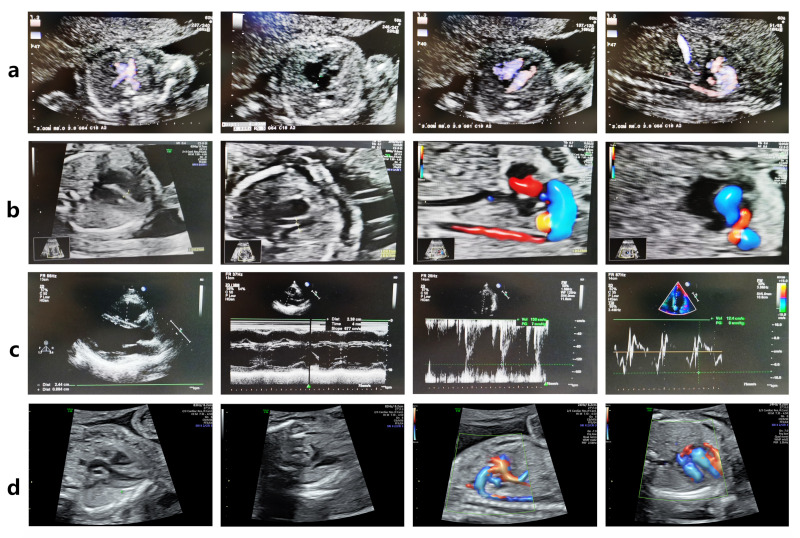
The *FLNC* variant leads to fetal Tetralogy of Fallot (TOF). (a) Fetal echocardiography at 22 weeks of gestation revealed TOF (pulmonary valve stenosis, ventricular septal defect, overriding aorta, and right ventricular hypertrophy) in the *FLNC* variant-carrying fetus IV-3. (b) Fetal echocardiography at 20 + 3 weeks of gestation revealed TOF in the proband IV-4. (c) The woman III-7 carries the *FLNC* variant but has a normal echocardiogram. (d) The fetus IV-5, who does not carry the *FLNC* variant, exhibited a normal echocardiogram at 23 weeks of gestation.
